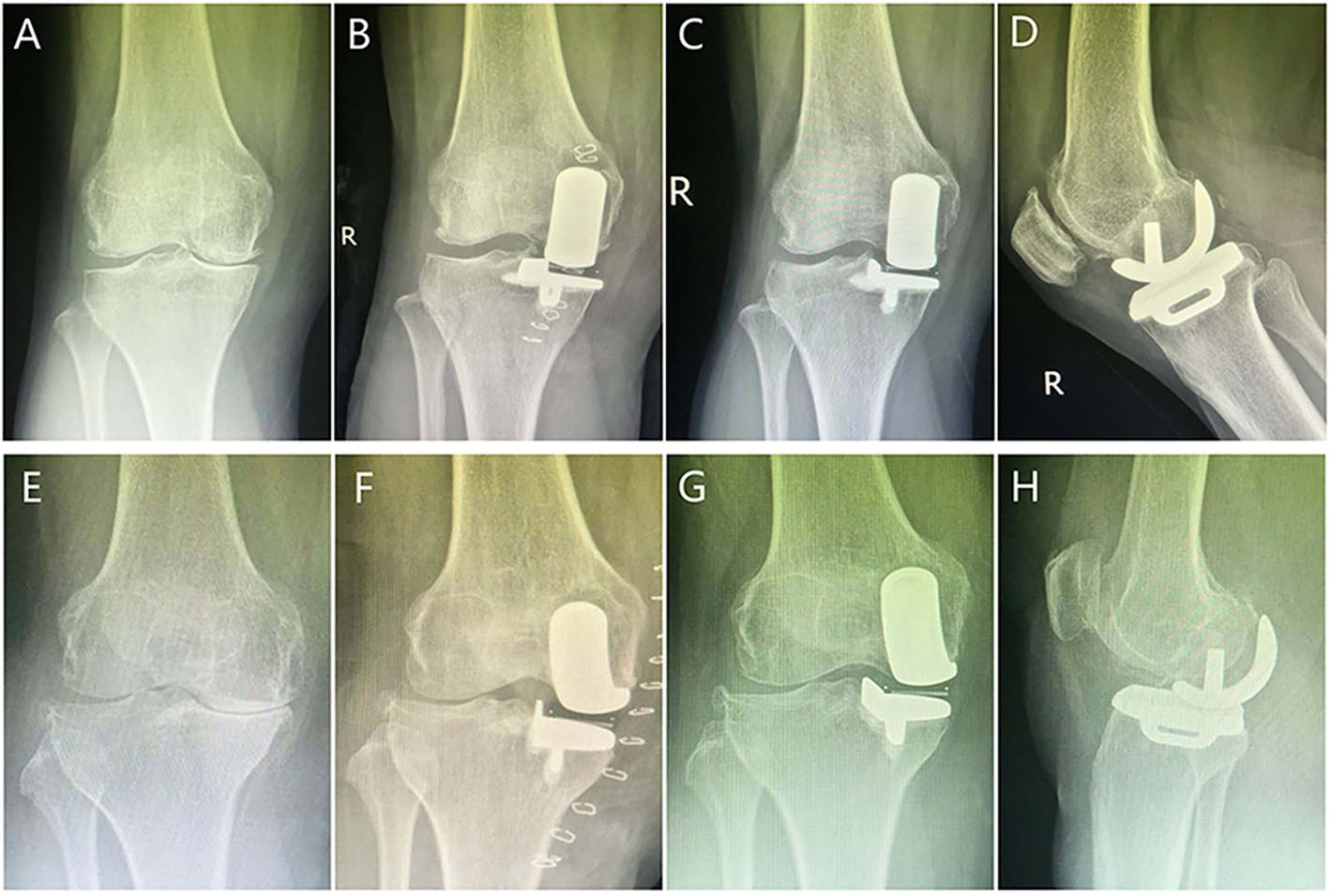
Radiographs of (A-D) a 54-year-old female patient and (E-H) a 69-year-old male patient with isolated compartment OA who underwent Oxford UKA and showed excellent outcomes at 5-year follow-up
